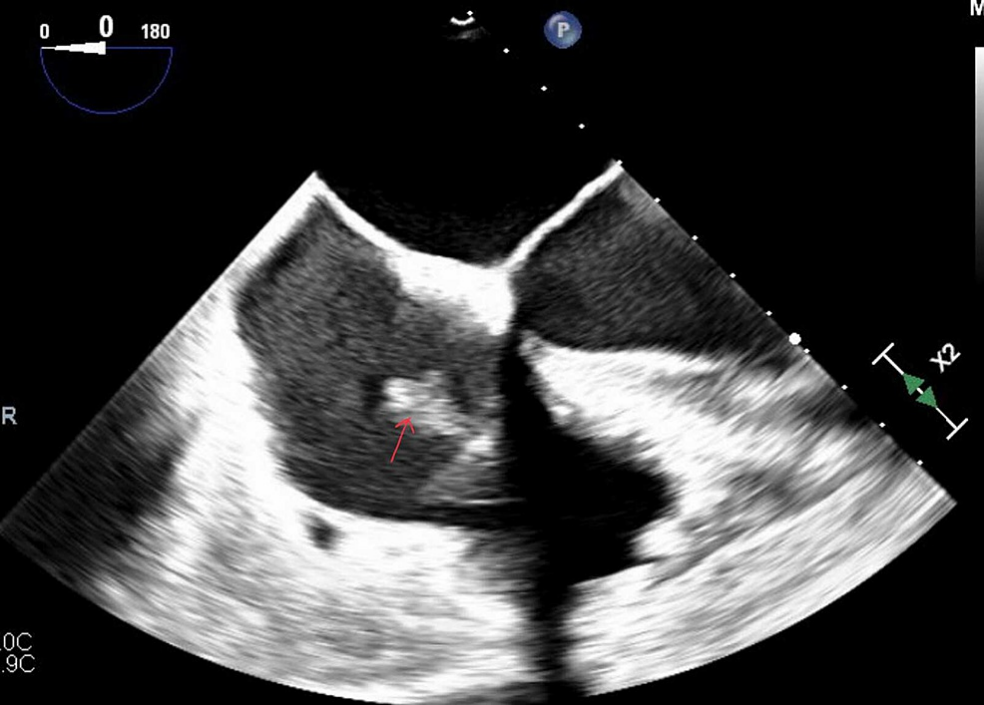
TEE showing the vegetations on tricuspid valve: 3 × 1.5 cm and 1.5 × 1.2 cm TEE: transesophageal echocardiography
